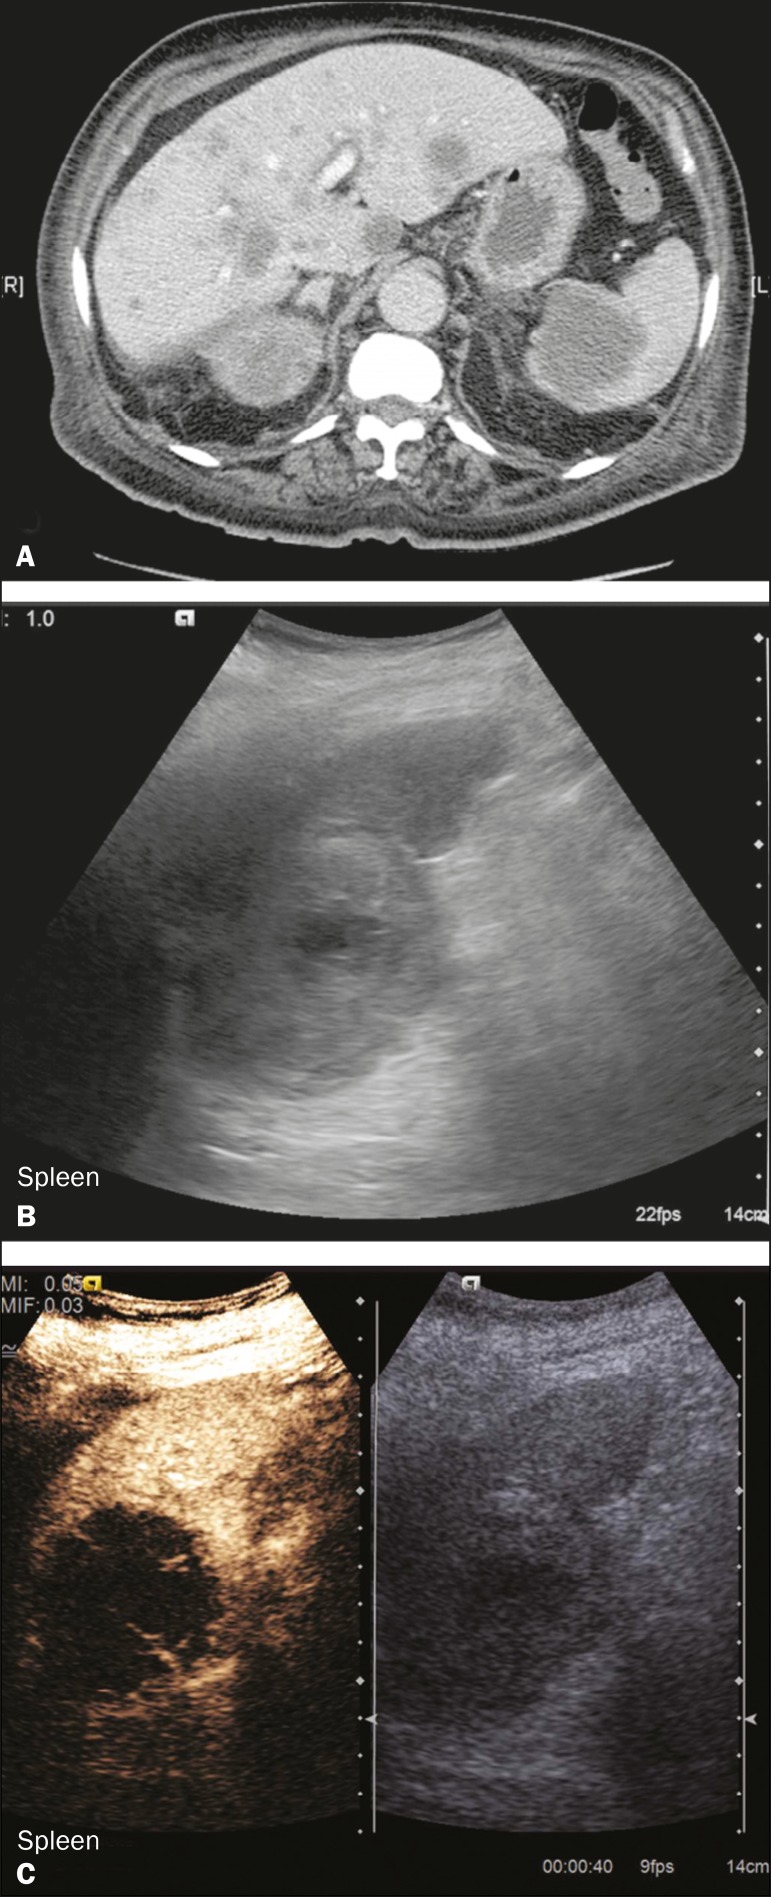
Splenic metastasis. A: CT scan showing several hypodense
